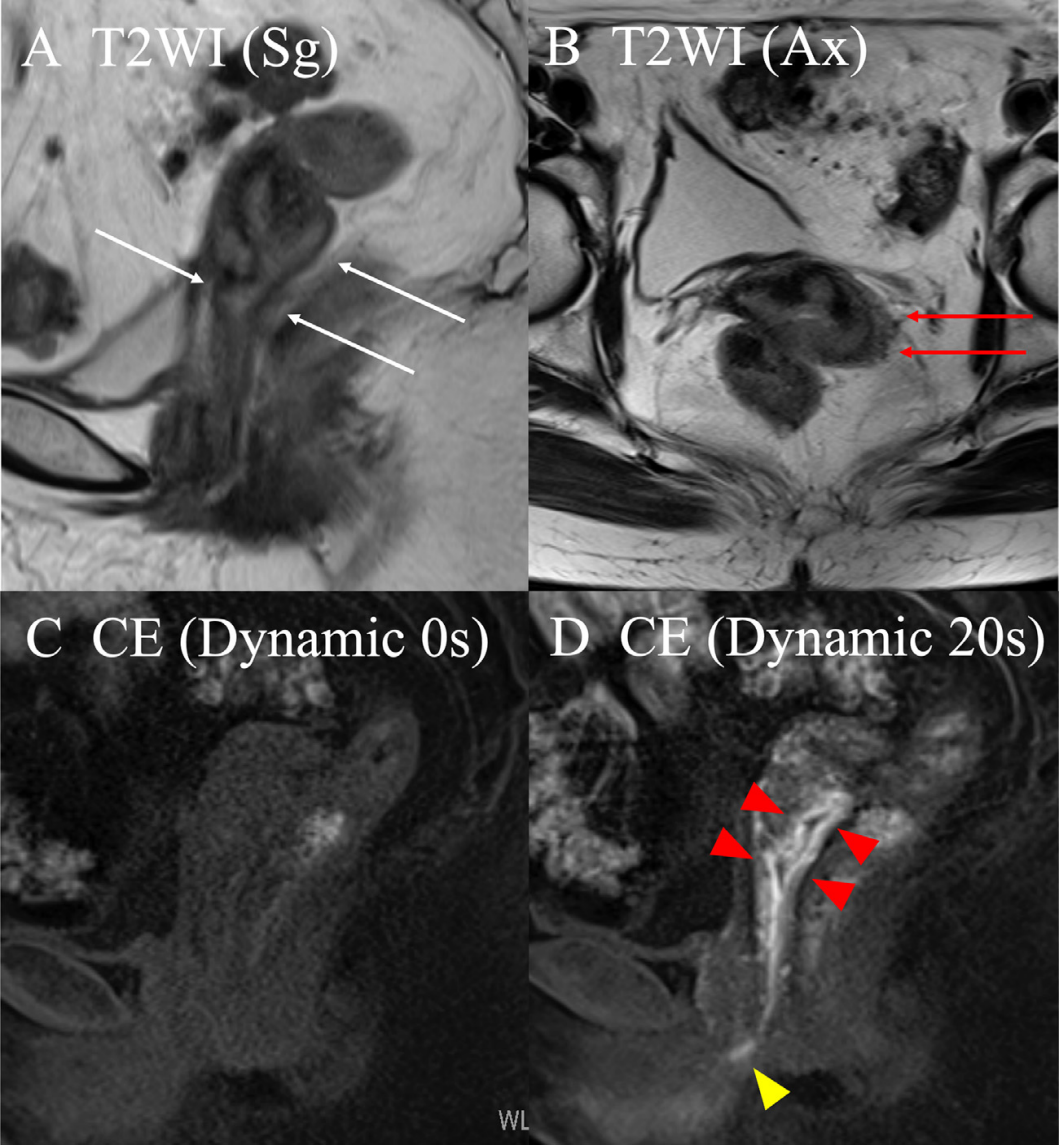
Pretreatment magnetic resonance imaging (MRI) findings of the cervix, vagina, and external urethral orifice. Sagittal (A) and axial (B) T2-weighted image of the cervix show tumor- extended vagina (white arrow) and small left parametrium invasion (red arrow). Predynamic enhanced sagittal T1-weighted image (C) and 20-seconds dynamic image (D) show early contrast effect in the cervix and vagina (red arrowhead) and external urethral orifice (yellow arrowhead).
